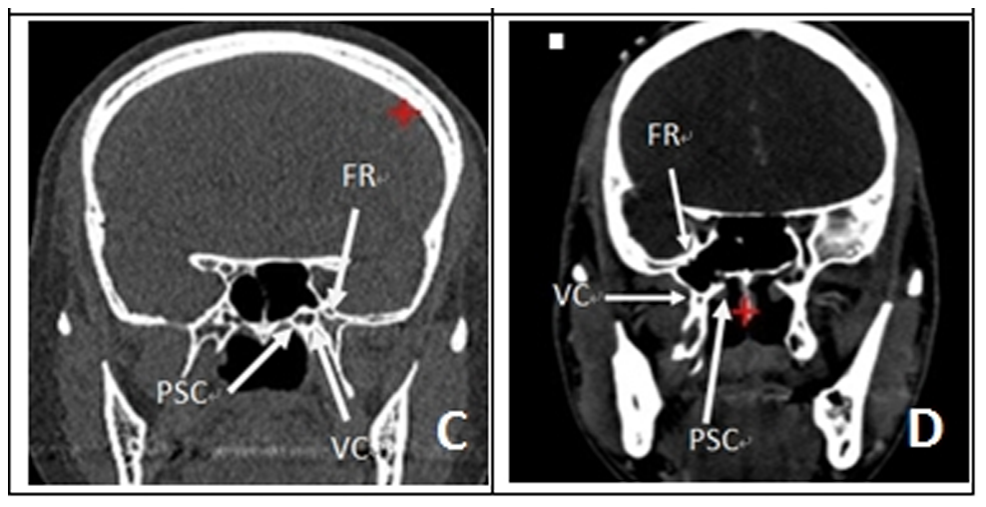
(C&D) Coronal CT examinations. it showed their relationship. (FR: foramen rotundum; PSC: Palatosphenoidal Canal (Palatovaginal Canal); VC: vidian nerve).
